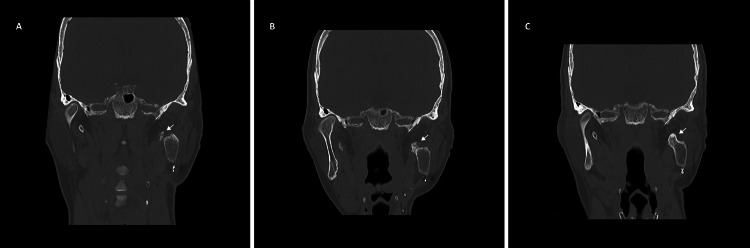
Postoperative coronal CT bone window (A) Minimally displaced fracture in the distal third of the left temporal styloid fracture, in proximity with the bone flap (white arrow). (B) Pseudoarthrosis of the left temporal styloid process in conjunction with the bone flap and concomitant hypertrophy of the bone fragments (white arrow). (C) Complete fusion of the left temporal styloid process with the bone flap (white arrow).
